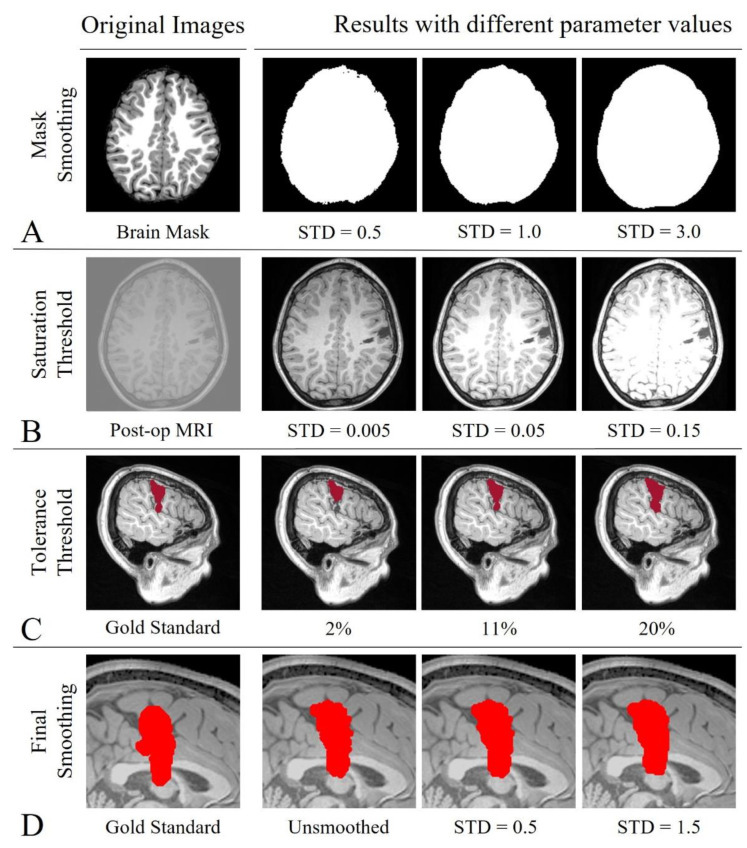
Results from low (2nd column) to high (4th column) parameter values. Mask Smoothing (A-1st row): original preoperative MRI brain mask (left) and three different binarized smoothed masks (right); Saturation Threshold (B-2nd row): low-contrast original postoperative MRI (left) and three contrast-adjusted images (right); Tolerance Threshold (C-3rd row): manual traced resection (left) and three examples of resections obtained with increasing tolerance value (right); and Final Smoothing (D-4th row): manual traced resection (left) and three different values of smoothing.
